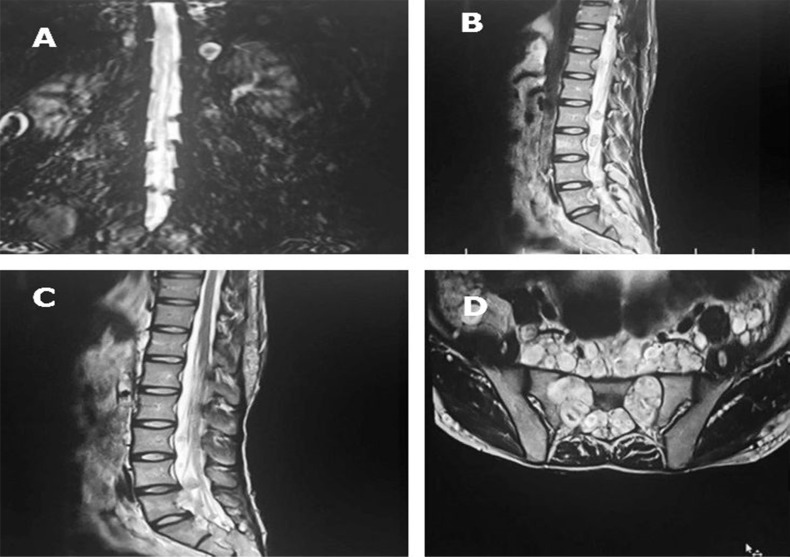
Widespread neurofibromas in lumbosacral nerve roots; In coronal magnetic resonance myelogram, multiple intradural neurofibromas arising from lumbosacral nerve roots are seen (A), Sagittal T2- weighted magnetic resonance imaging (MRI) of lumbosacral spine shows neurofibromas arising from nerve roots (B and C), Axial T2-weighted MRI demonstrates innumerable neurofibromas in the presacral space and in the sacral neural foramina causing enlargement of corresponding neural foramina (D).
